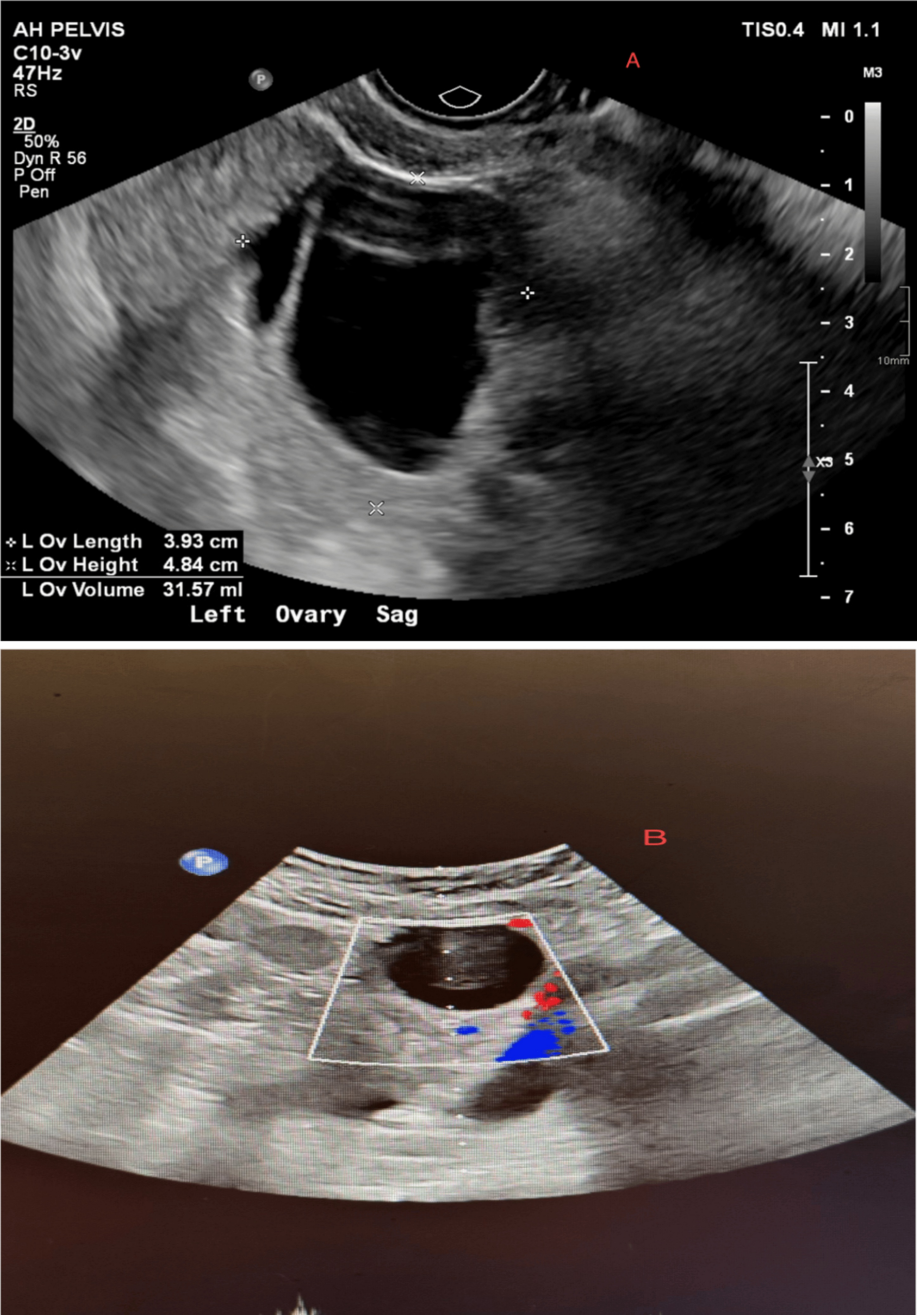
The left ovary was enlarged with a size of 3.8 × 3.2 × 3.9 cm (A). Doppler ultrasound (B) depicting diminished arterial and venous flow to the left ovary for Patient B, due to the potential 4-cm anechoic cyst shown in the upper right of the image.
